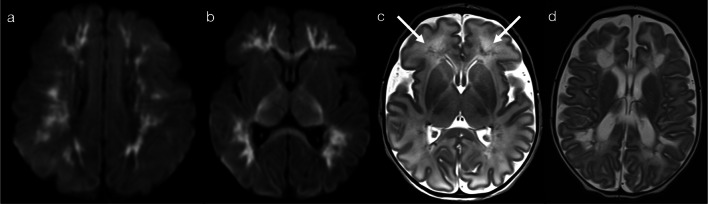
Restricted diffusion in supratentorial white matter—diffuse (Pattern 1A). 2-day-old term infant with generalised seizures and CSF-proven parechovirus encephalitis. DWI (a, b) demonstrated a striking pattern of restricted diffusion involving deep and periventricular white matter. On T2-weighted imaging (c), corresponding foci of low signal intensity were relatively subtle (arrows). A follow-up scan 5 weeks later (d) revealed significant tissue loss with areas of cystic encephalomalacia
